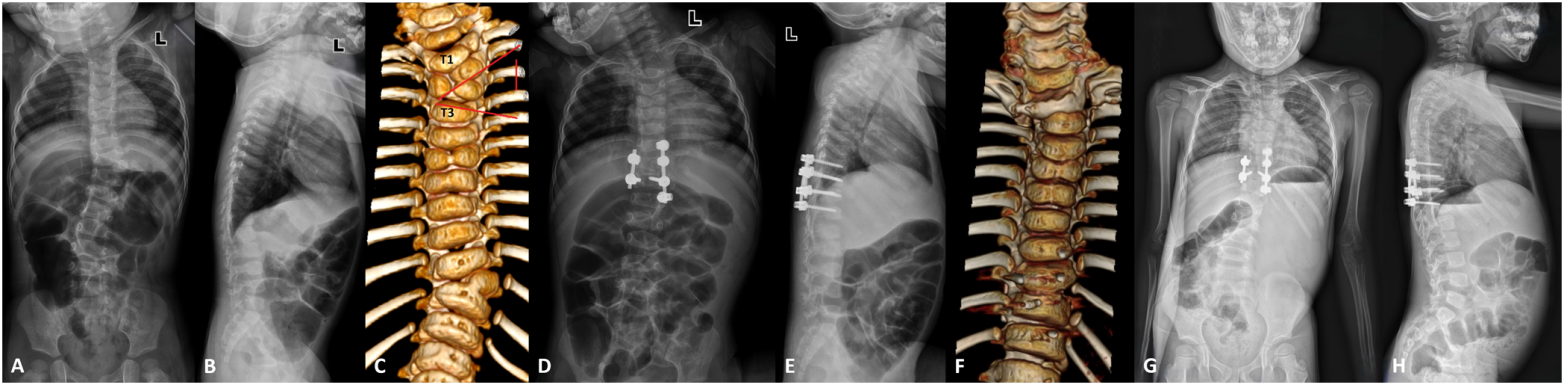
A 1-year-old girl (case 27) with congenital cervicothoracic scoliosis. (A, B) Preoperative radiographs showed that the segmental scoliosis was 43°, the distal compensatory curve was 23°, the T1 tilt was 26°, the clavicle angle was 10°, and the neck tilt was 20°. (C) Preoperative CT three-dimensional reconstruction showed the left T1-T2 hemivertebra and the T2 butterfly vertebra. (D,E) Posterior T2 left hemivertebra resection without internal fixation was performed. Postoperative radiographs showed that the segmental scoliosis was 16° with a postoperative correction rate of 62.8%, the distal compensatory curve was 5°, the T1 tilt was 17°, the clavicle angle was 7°, and the neck tilt was 15°. (F) Postoperative CT demonstrated a solid fusion. (G,H) At the 5-year follow-up, radiographs showed that the segmental scoliosis was 4° with a correction rate of 90.7%, the distal compensatory curve was 2°, the T1 tilt was 3°, the clavicle angle was 1°, and the neck tilt was 6°. No significant loss of correction was observed during the follow-up.
